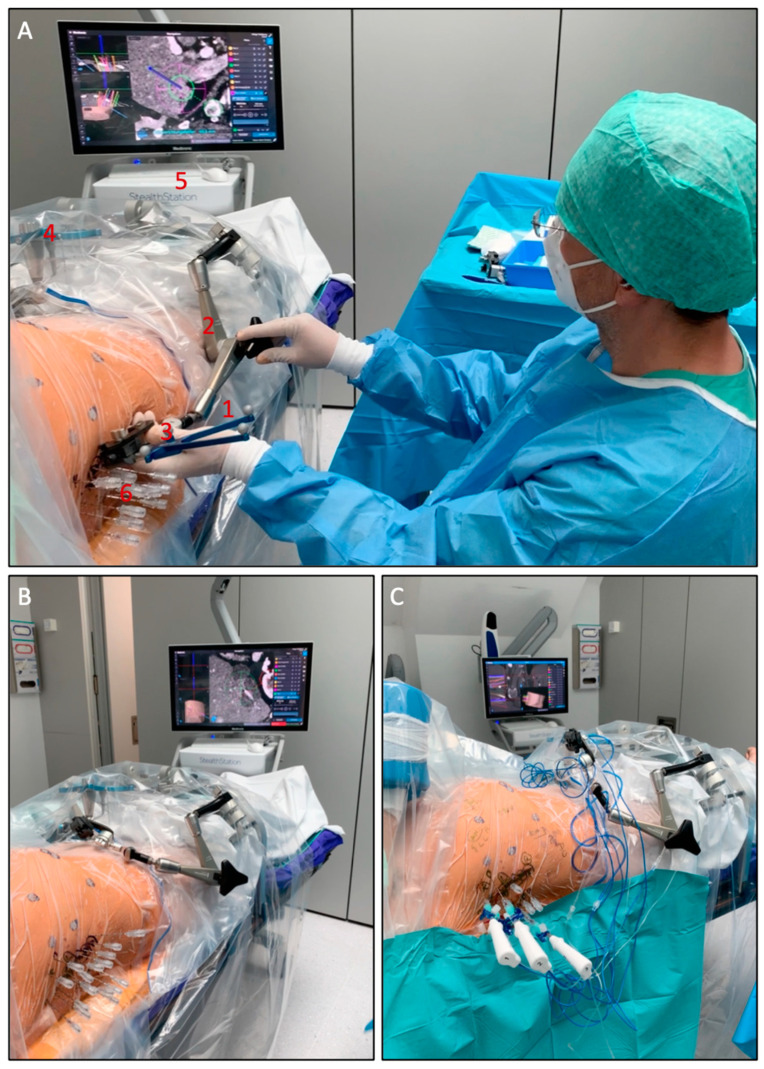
Setup of the SRFA procedure. (A) The probe (1) of the navigation system is inserted into the ATLAS aiming device (2). The reflective markers (3) on the probe and the reference frame (4) are tracked by the camera of the navigation system (5). After manual alignment of the aiming device with the virtual trajectory and consecutive removal of the probe, the interventionalist introduces several coaxial needles (6) through the locked aiming device to the preplanned target point. (B) Placed coaxial needles in final position. (C) After verification of correct needle placement with a non-enhanced control CT scan and image fusion with the planning-CT up to three RF-electrodes at a time are introduced through the coaxial needles for serial tumor ablation.
